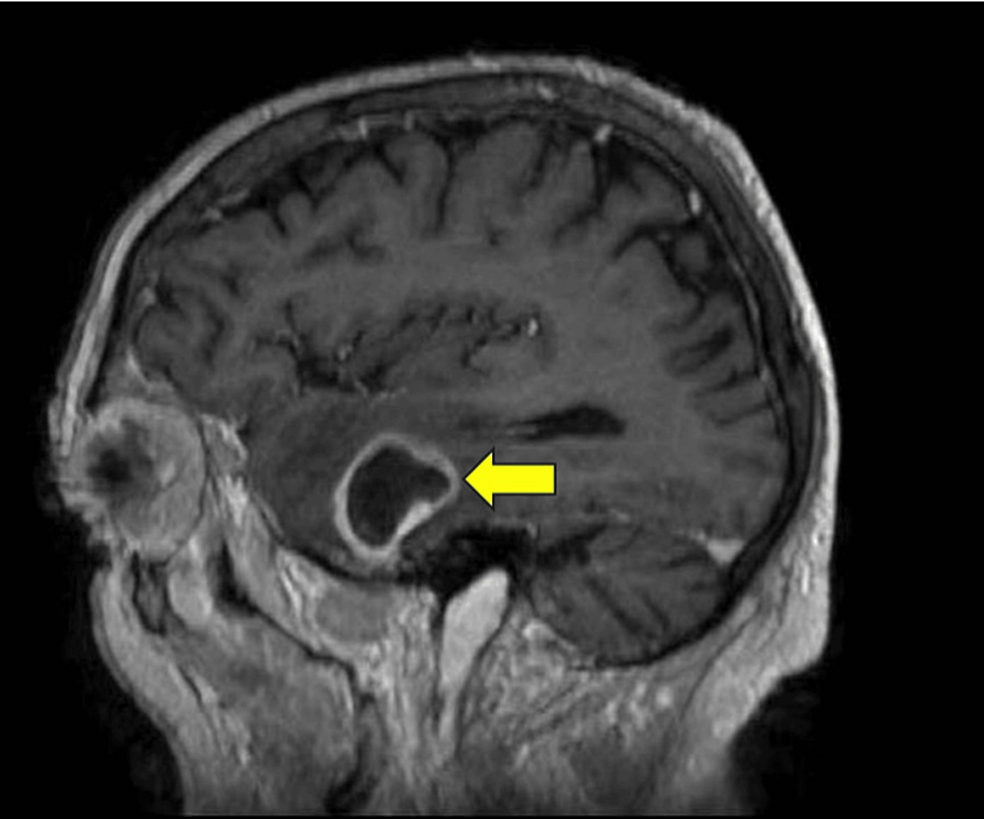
Sagittal plane Contrast-enhanced magnetic resonance imaging of the head shows a ring-enhancing lesion (yellow arrow) in the left temporal lobe with a central hypointense area (cystic) and a thick rim, along with surrounding regional edema of the temporal lobe.
